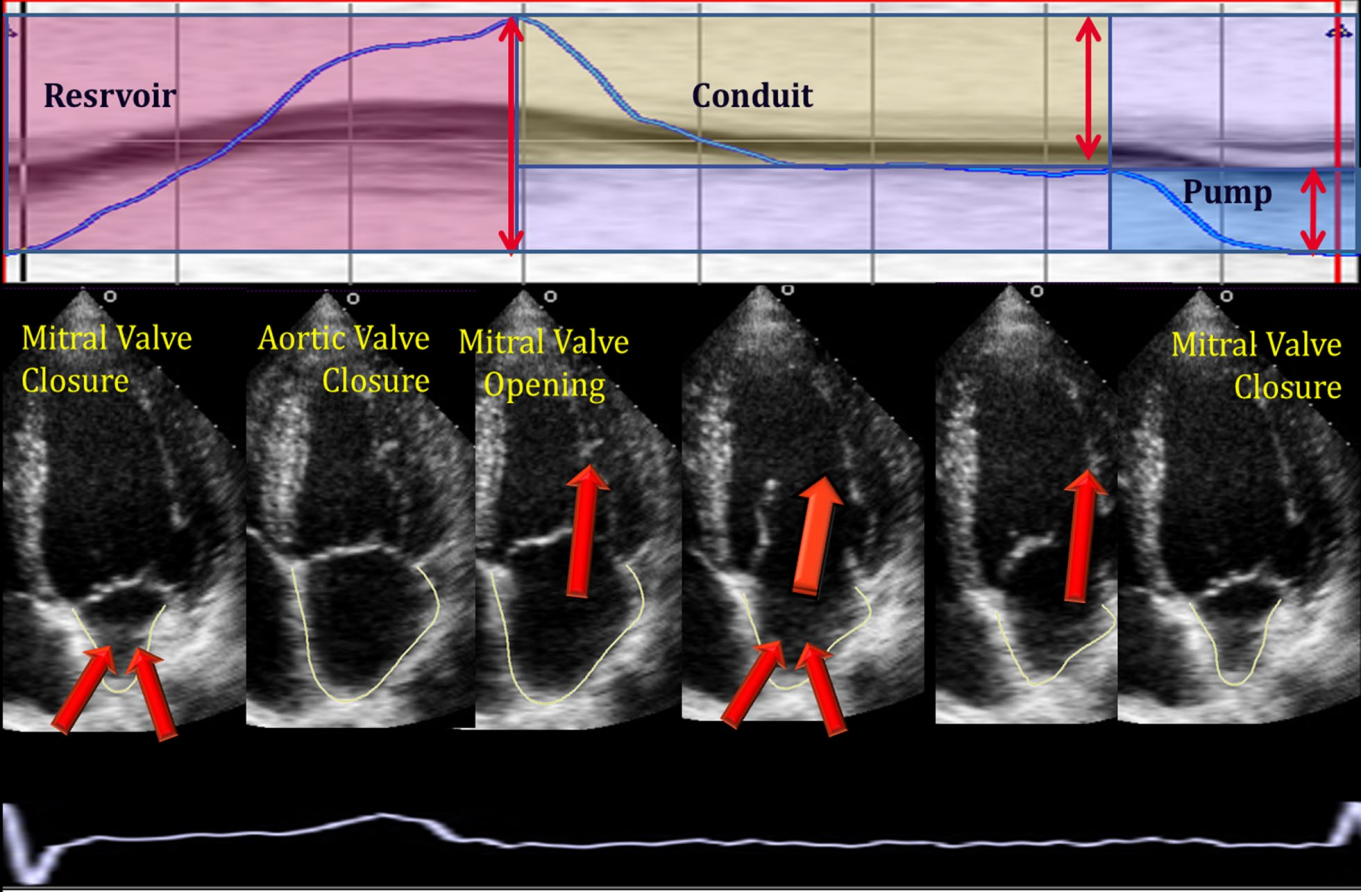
Left Atrial volumes and function, and the cardiac cycle acquired automatically by the VVI software following endocardial surface manual tracing using a point and click approach as seen in a patient with sinus rhythm on echocardiography
